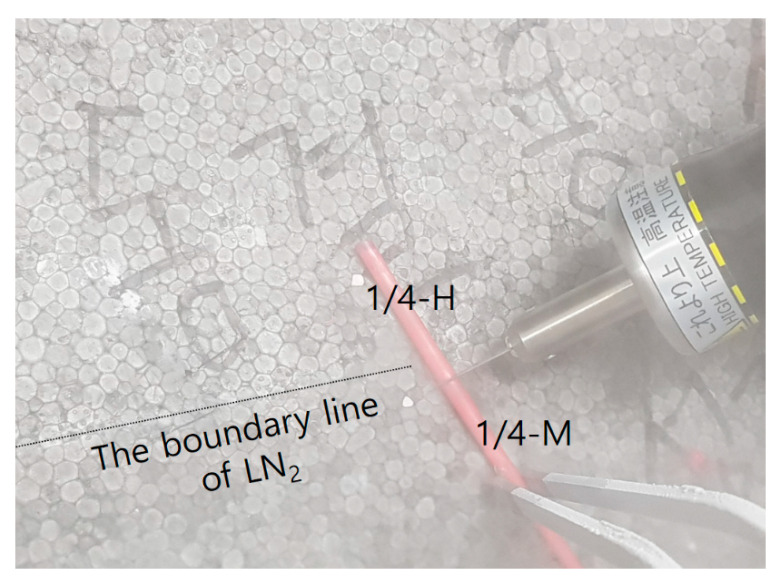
An image of the ultrasonic cutter on the frozen semen straw. The bottom of the tilted Styrofoam box was used to expose the cutting area of frozen semen to the surface of LN2.
